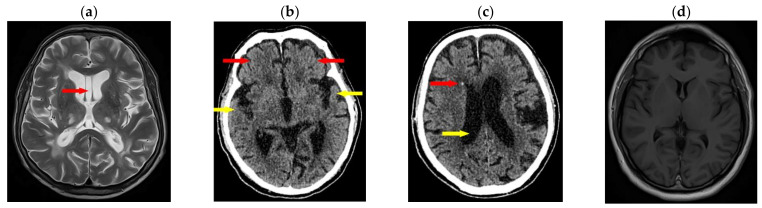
Typical findings in chronic traumatic encephalopathy: (a) A 58-year-old male with a history of repetitive trauma MRI in T2 sequence; cave of septum pellucidum (red arrow) is visible between enlarged lateral ventricles, (b) Advanced atrophy of frontal and temporal lobes (red and yellow arrows respectively) visible in plain CT scans, (c) Enlargement of ventricles in atrophied brain (yellow arrow); calcification (red arrow), suggestive of a neurodegenerative process, (d) MRI of a healthy person for reference.
